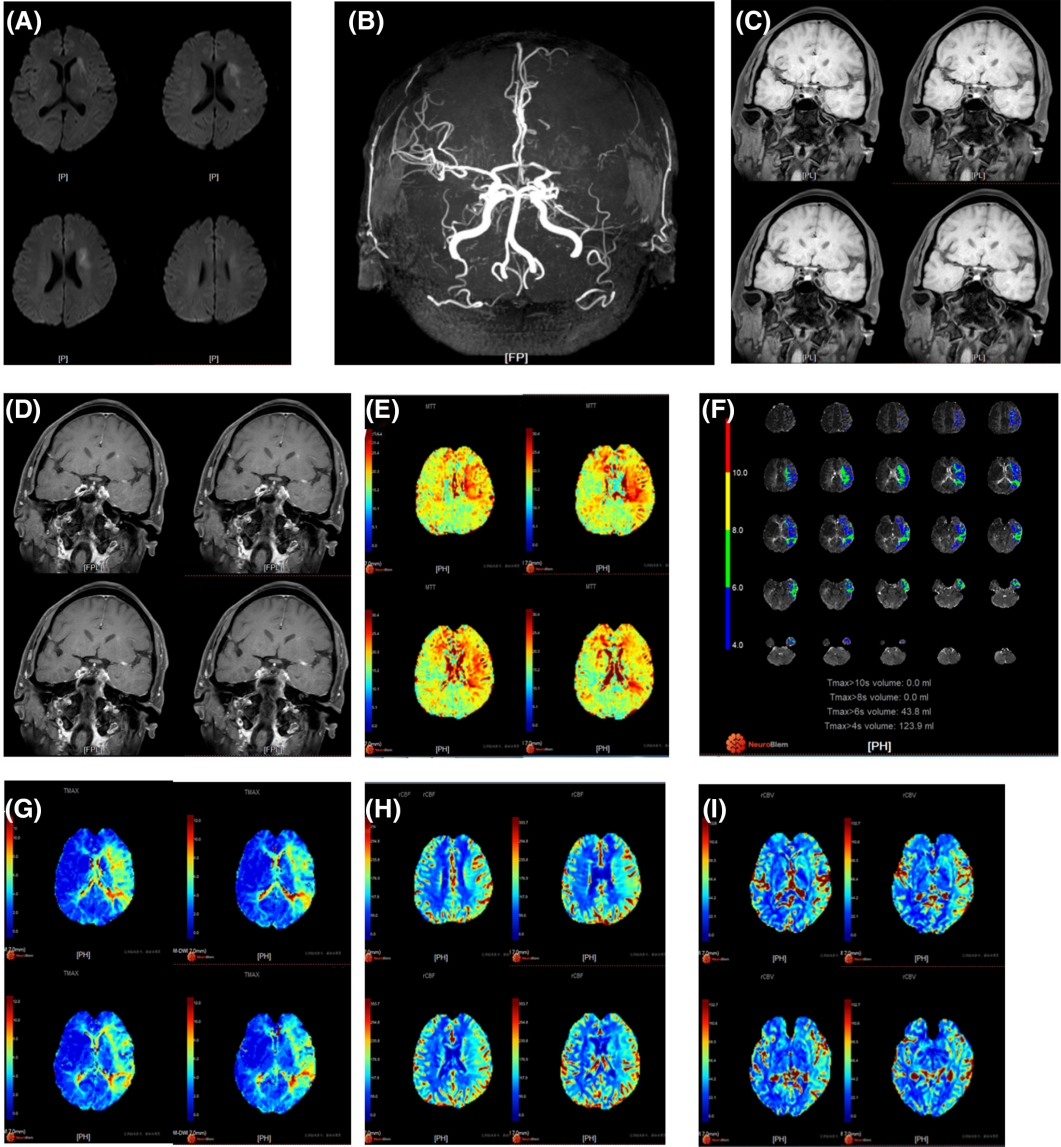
MRA, High‐resolution Vessel Wall and Magnetic Resonance perfusion measurement of non acute middle cerebral artery occlusion (NAMCAO) patients. (A) DWI showed the cerebral infarction of the patient. (B) MRA showed the occluded Left‐MCA of the patient. (C, D) High‐resolution vessel wall of the patient showed the occlusion site and length. MTT (E), Tmax (F, G), CBF (H), CBV (I) showed the perfusion condition of the patient.
